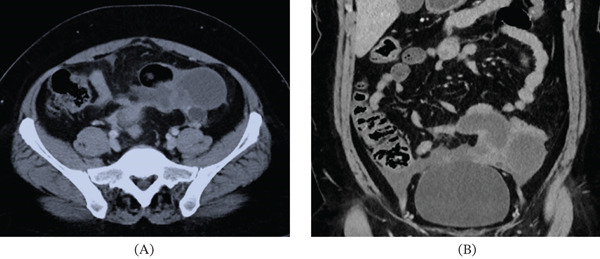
The (A) axial and (B) coronal CT findings on the patient including a large complex left adnexal cyst measured at 10.1 × 7.0 × 9.3 cm and moderate free peritoneal fluid.
